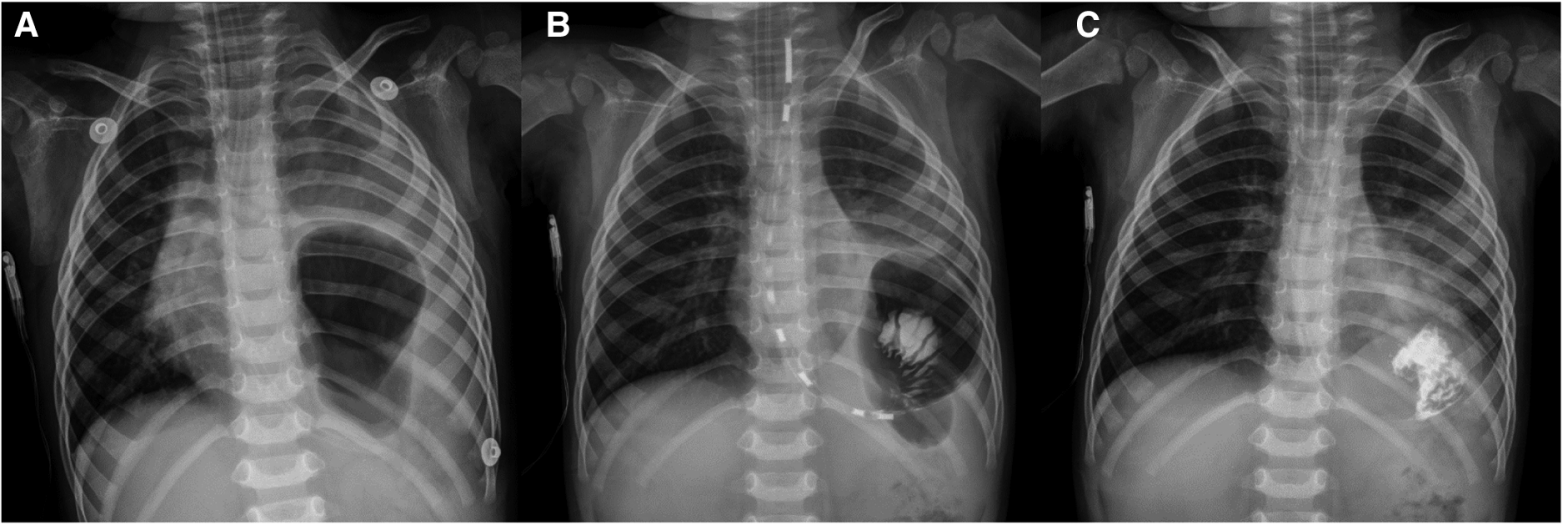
Case 3: x-ray manifestations before and after operation: (A) encapsulated gas accumulation with mediastinal displacement; (B) the gastric tube is not bent, and its distal end is located in the stomach; (C) after adjusting the gastric tube for the complete decompression, there was no encapsulated gas accumulation with mediastinal displacement.
